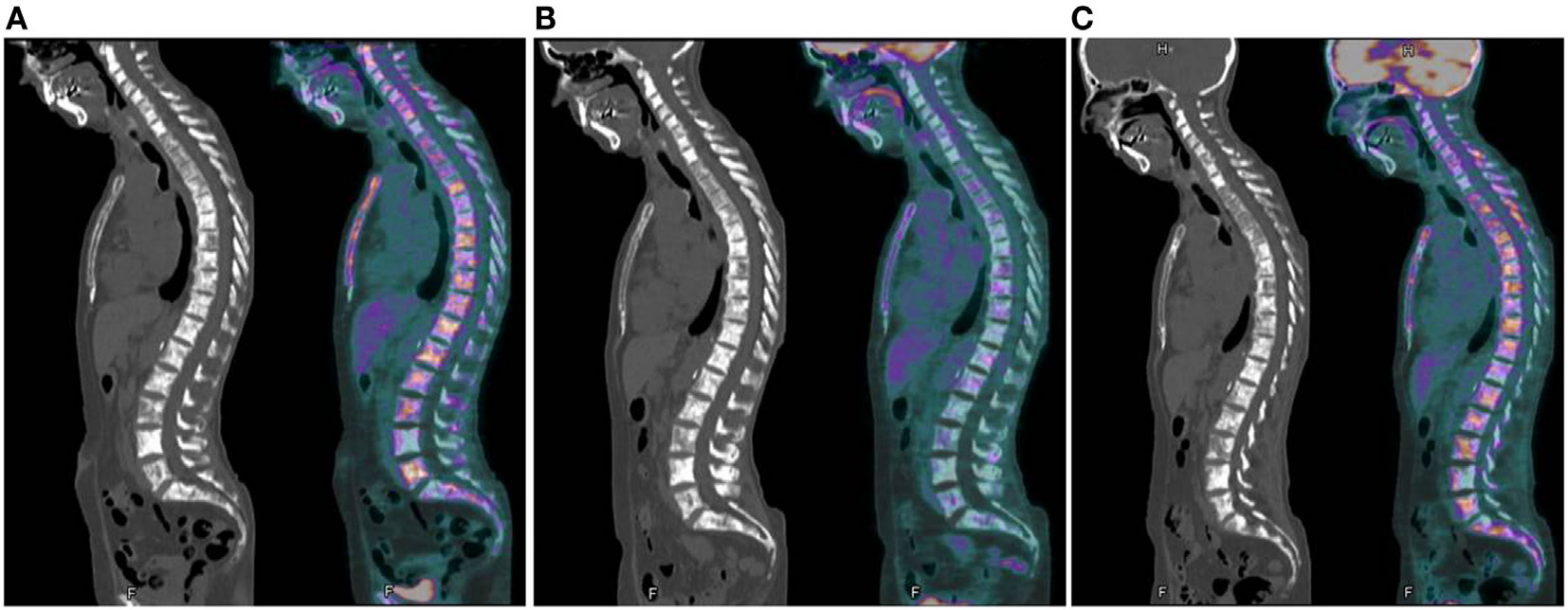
Example of metabolic assessment in a patient with metastatic bone evolution. (A) First examination: initial evaluation with multiple bone lesions (SUVmax = 11.8; SUVpeak = 7.1); (B) second examination: partial metabolic response on bone (SUVmax = 3.4 or 71% decrease; SUVpeak = 1.5 or 78% decrease); and (C) third examination: disease progression with new lesions and recurrence of some initial hypermetabolic lesions (SUVmax = 6.4 or 46% increase; SUVpeak = 4.3 or 65% increase). Persistence of sclerosis on all CT images does not allow to evaluate the response.
